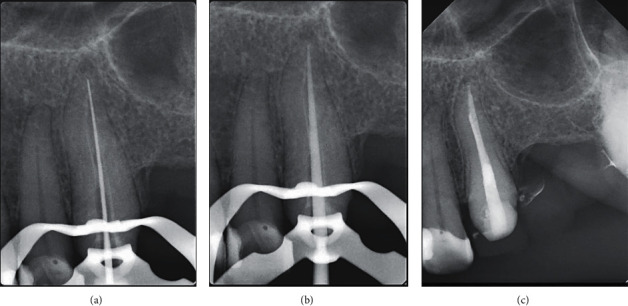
(a) Working length determination radiograph. (b) Master gutta-percha cone radiograph. (c) Final radiograph.
